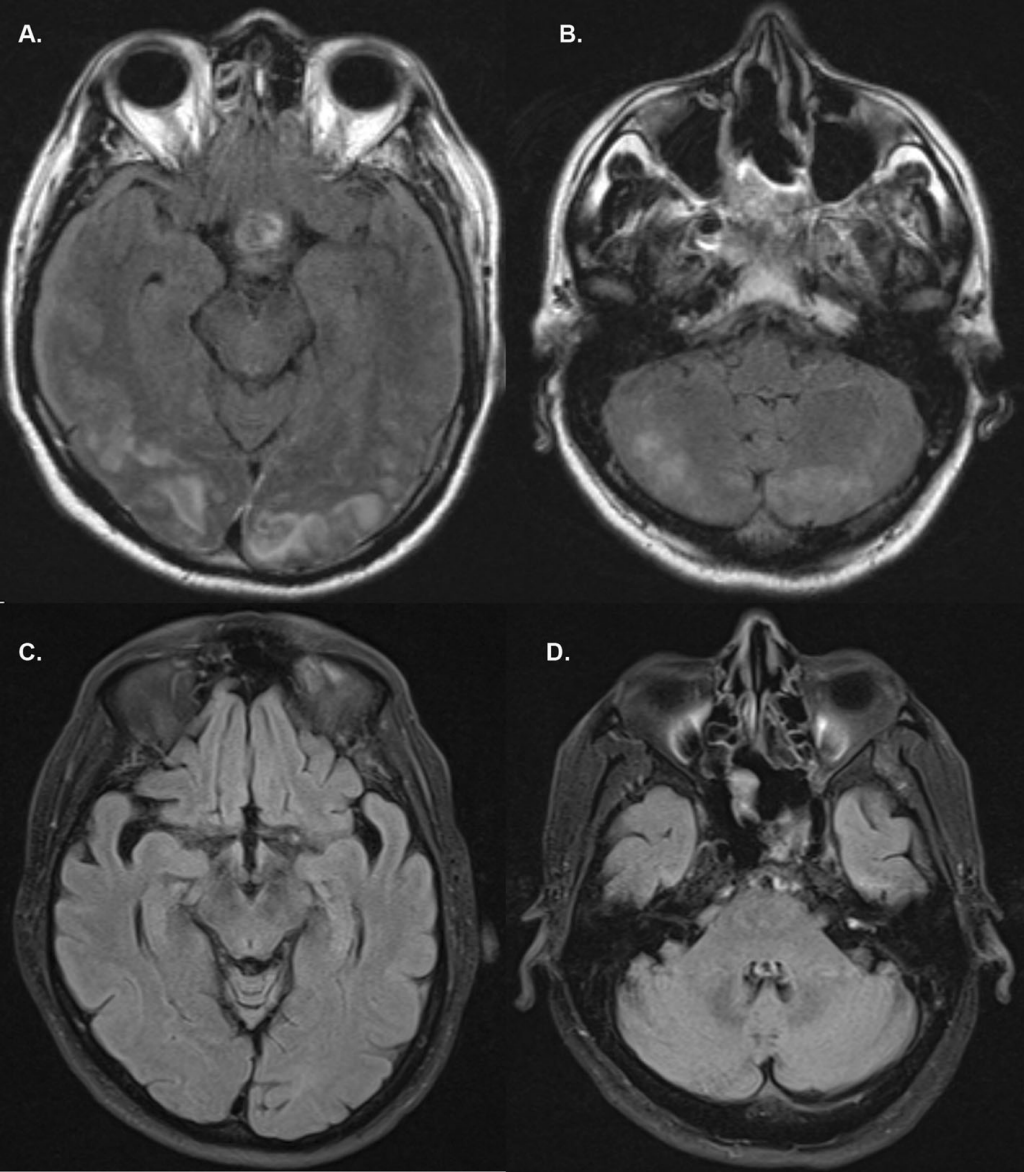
Axial brain MRI reveals patchy areas of increased T2 FLAIR hyper-intensity in the bilateral occipital lobes (a) and posterior cerebellum (b). Follow-up MRI at 6-months follow-up show complete resolution of the areas of T2 FLAIR hyper-intensity in the bilateral occipital lobes (c) and posterior cerebellum (d)
